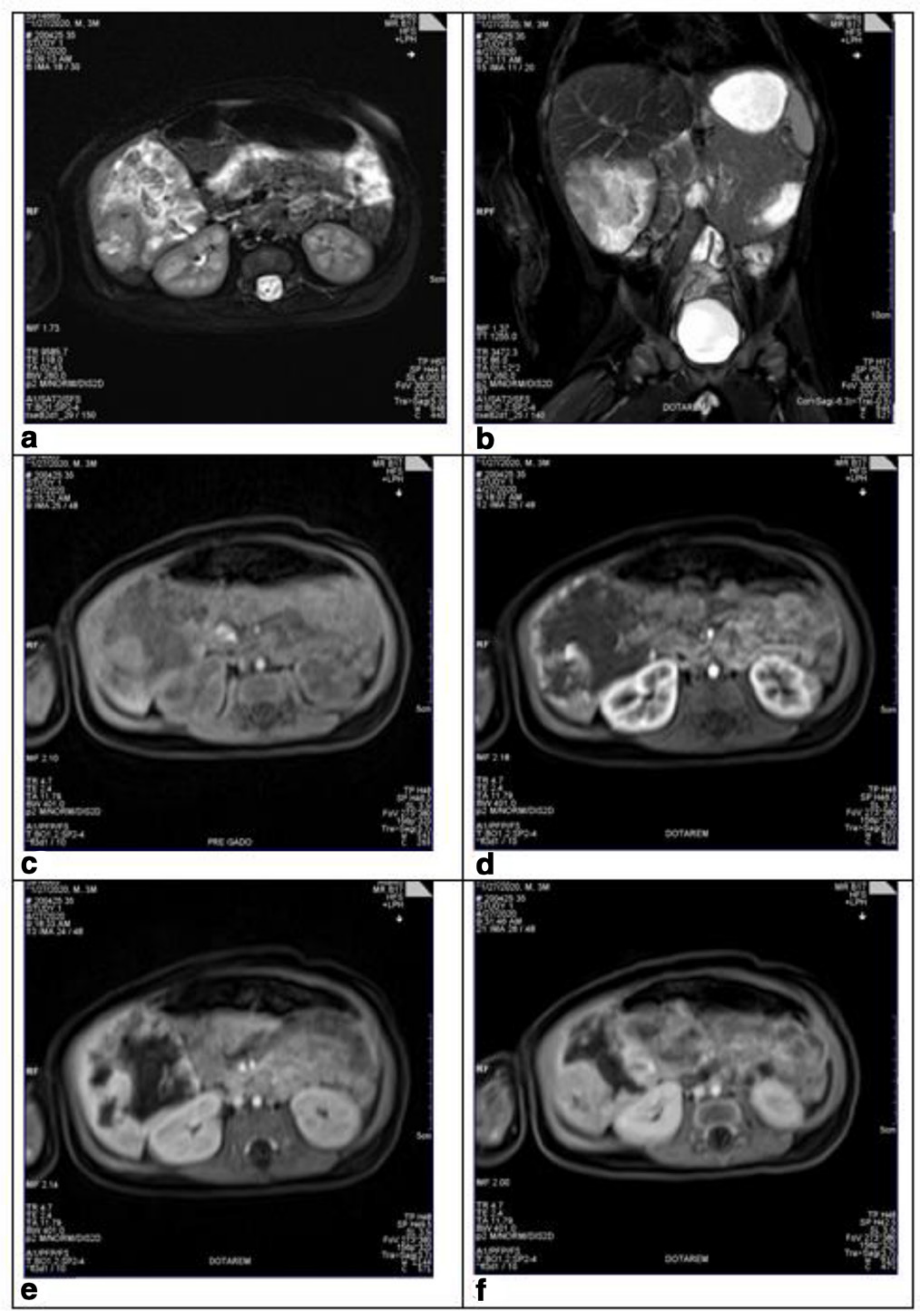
Contrast-enhanced MRI detected a right hepatic lesion in the segment VI. (a and b) The lesion had heterogeneous high signal intensity in axial and coronal T2W. c: The lesion has heterogeneous low signal intensity in T1W and T1 pre gadolinium. d: Heterogeneous gadolinium contrast opacification, peripheral enhancement of the arterial phase. (e and f) Contrast opacification progressive centripetally in the venous phase (T1 portal venous phase) and late phase (T1 delay phase) and without filling the whole lesion.
